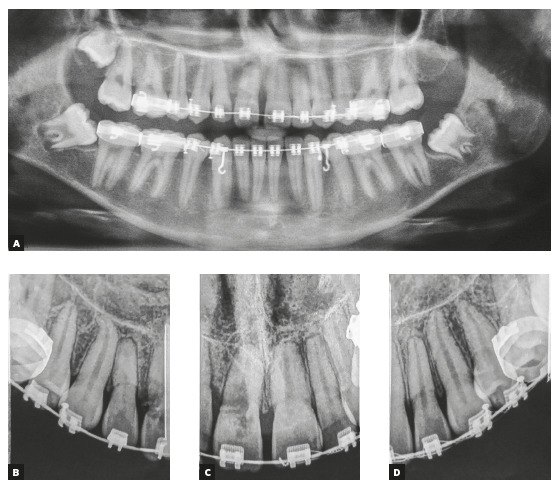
Routine panoramic and periapical radiographs requested after 17
